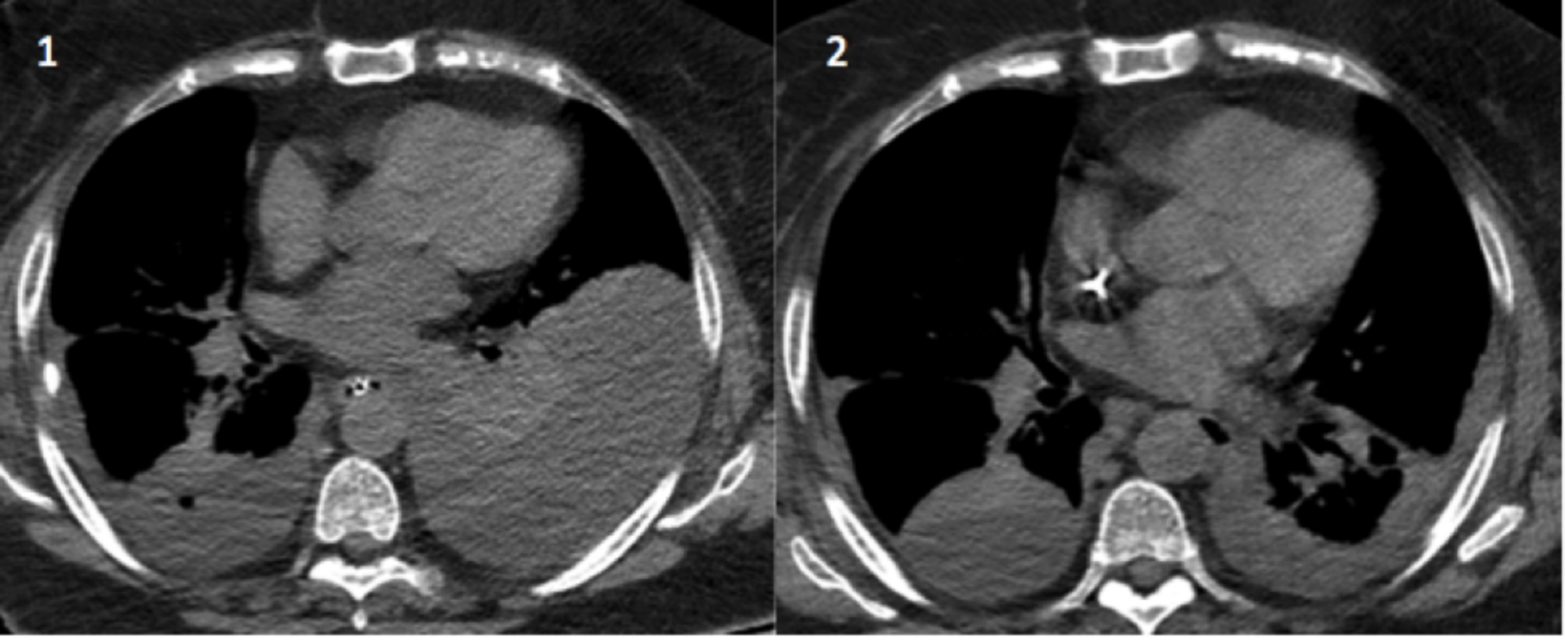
Chest CT scans Before (1) and after (2) chest computed tomography (CT) images following the administration of alteplase through the left chest tube into the pleural space
